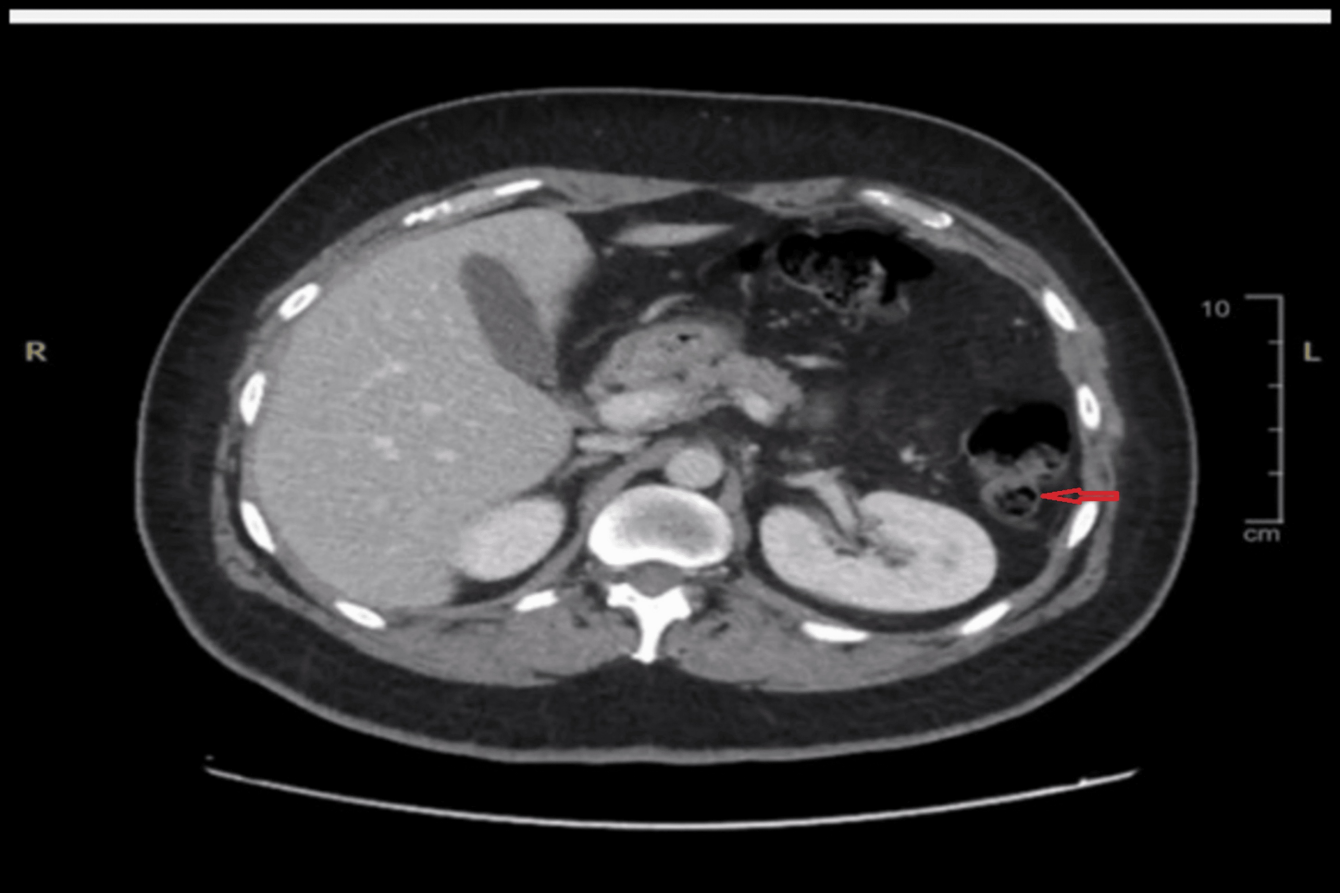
A computed tomography scan with contrast showing a focal diverticulum in the proximal/mid jejunal loop associated with diffuse wall thickening, significant surrounding fat stranding and adjacent pneumoperitoneum, and a small fluid collection.
